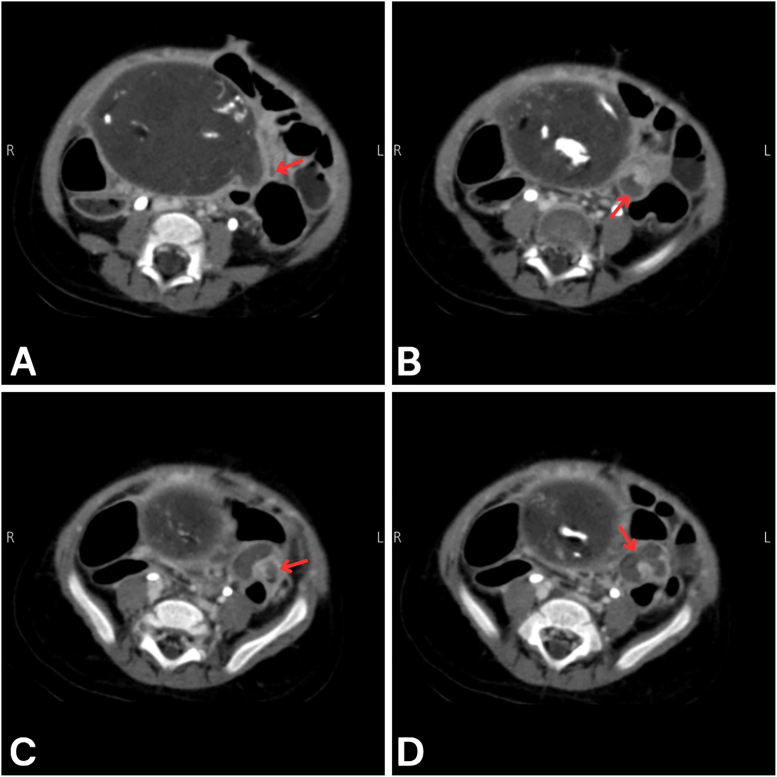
Sequential axial abdominal CT images with IV contrast showing the "whirlpool sign". A: The superior aspect of the twisted vascular pedicle (red arrow) appearing adjacent to the large cystic teratoma. B: Progression of the spermatic cord rotation (red arrow), beginning to form a spiral pattern. C: Further progression of the spermatic cord rotation (red arrow). D: The inferior extent of the twisted vascular pedicle (red arrow).
